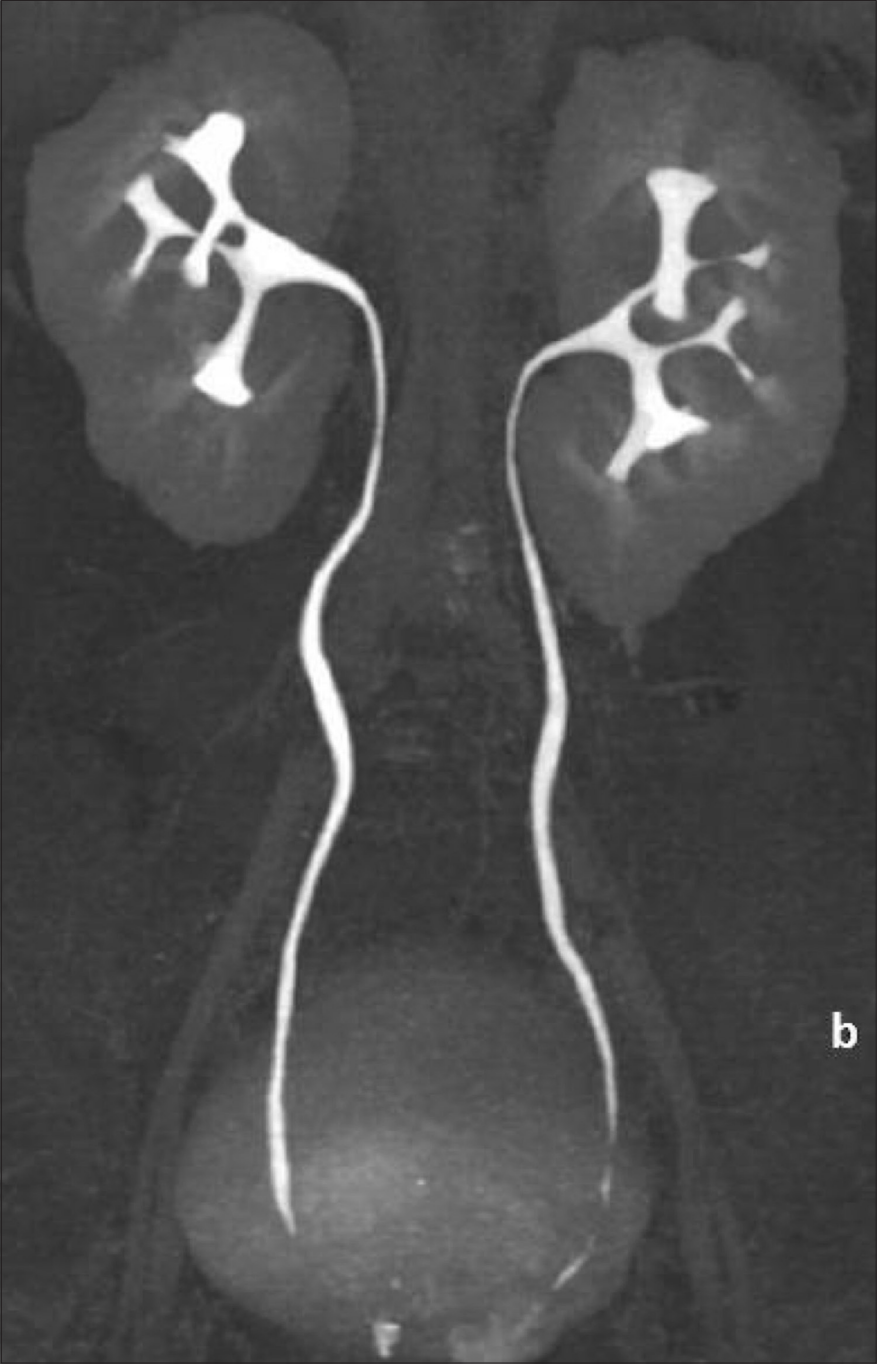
CT urogram. A maximum intensity projection, coronal CT scan image shows splayed and non-dilated excreting collecting systems
